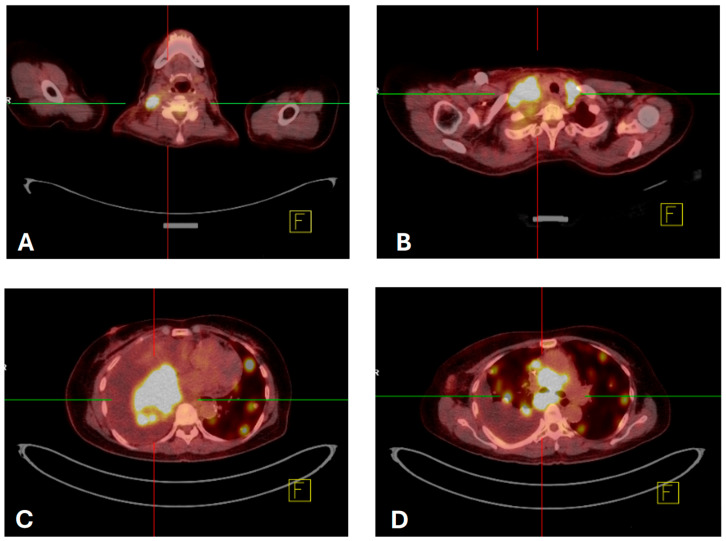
ET/CT scans of the patient showing high FDG uptake, mainly in the right lung with lung-to-lung and distant neck nodal metastasis, performed on 18 January 2024. Round high-density lesions can be observed in the following locations: (A) enlarged lymph nodes in the right neck, (B) enlarged lymph nodes in the bilateral supraclavicular fossa and mediastinum, (C) primary right lung tumor, and (D) diffuse round high-density lesions in both lungs, indicating lung-to-lung metastasis.
